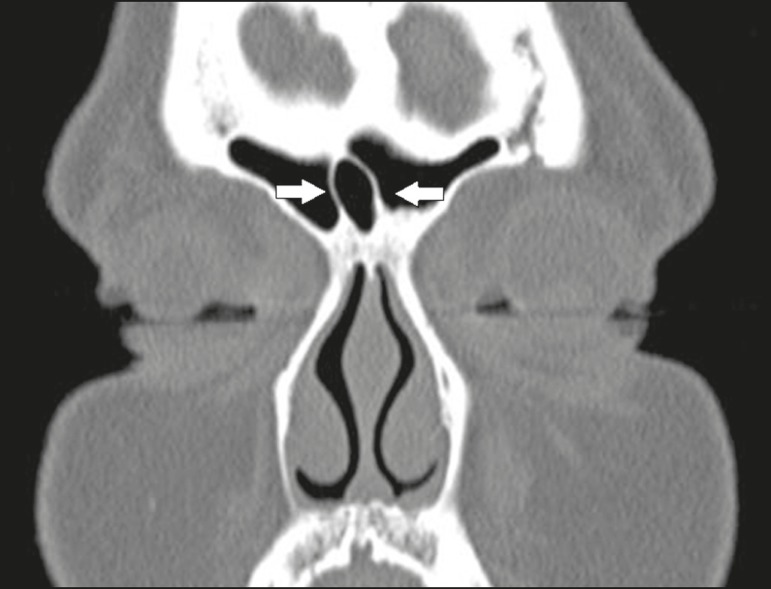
Coronal CT slice, with bone window settings, showing the frontal sinus
